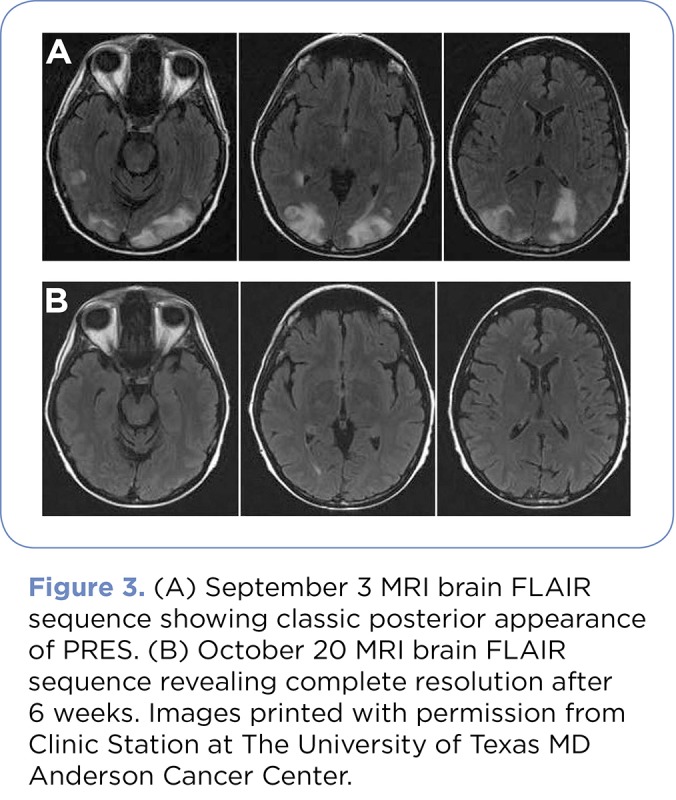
Figure 3. (A) September 3 MRI brain FLAIR sequence showing
